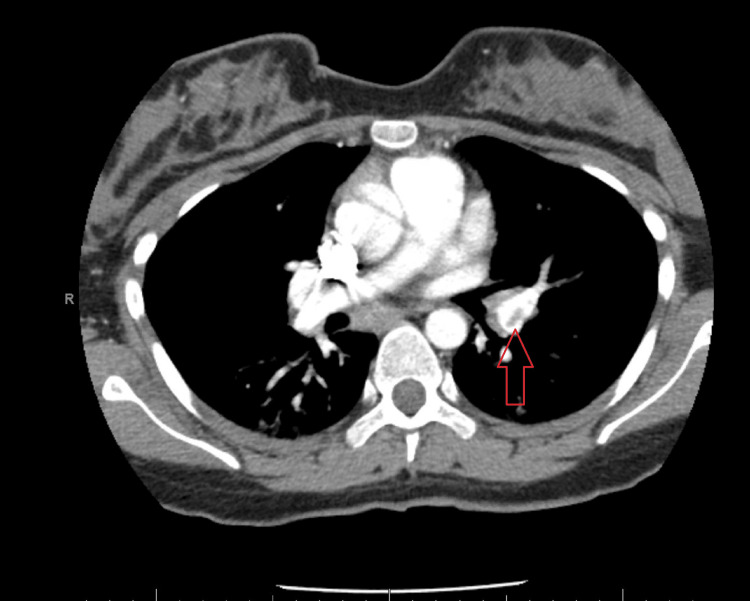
Patient's CT pulmonary angiogram. Red arrow: clot in the left pulmonary artery.
